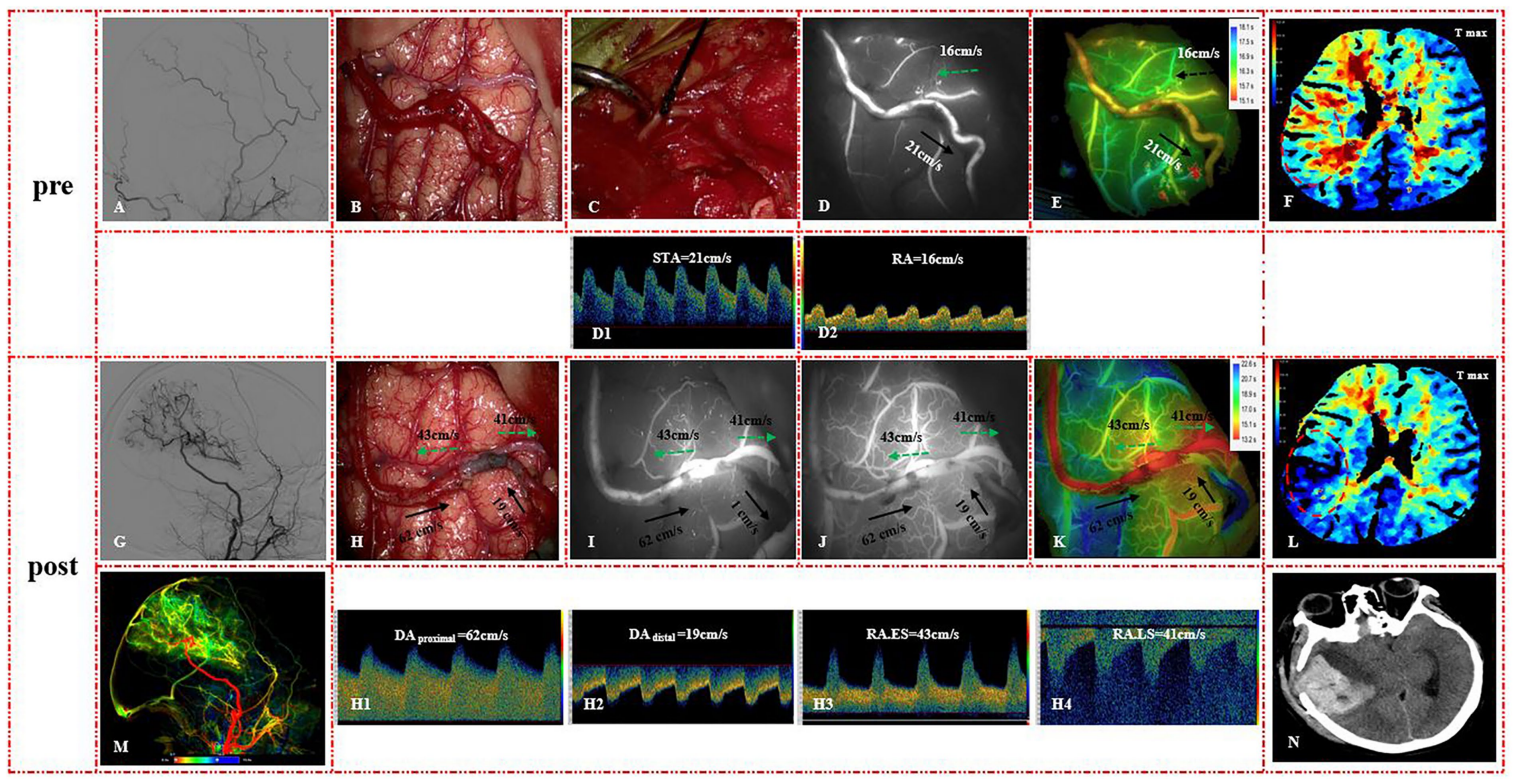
(A) Lateral view of the ECA on preoperative DSA. (B) Difficulty in anastomosis due to the distance between the STA and RA. (C) Ligation and transection of the frontal branch of the STA. (D–F) ICG angiography, Flow 800, and CT perfusion images before the bypass. (D1,D2) MVV of the STA and RA before the bypass. (G,M) Postoperative DSA demonstrated bypass patency. (H) MDU results after the bypass. The MVV of DA_proximal_
